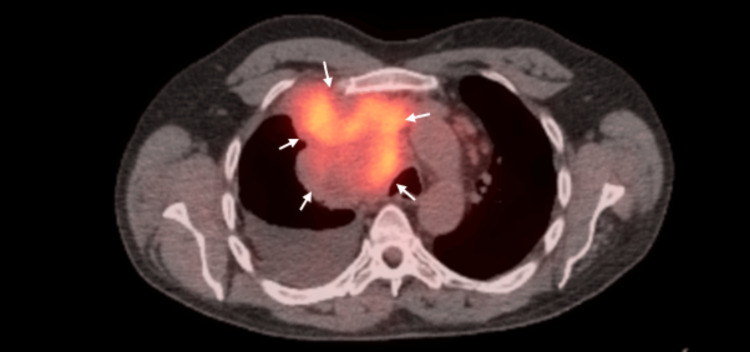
PET/CT showing a hypermetabolic mediastinal mass as indicated by the arrows. PET/CT: positron emission tomography/computed tomography.
